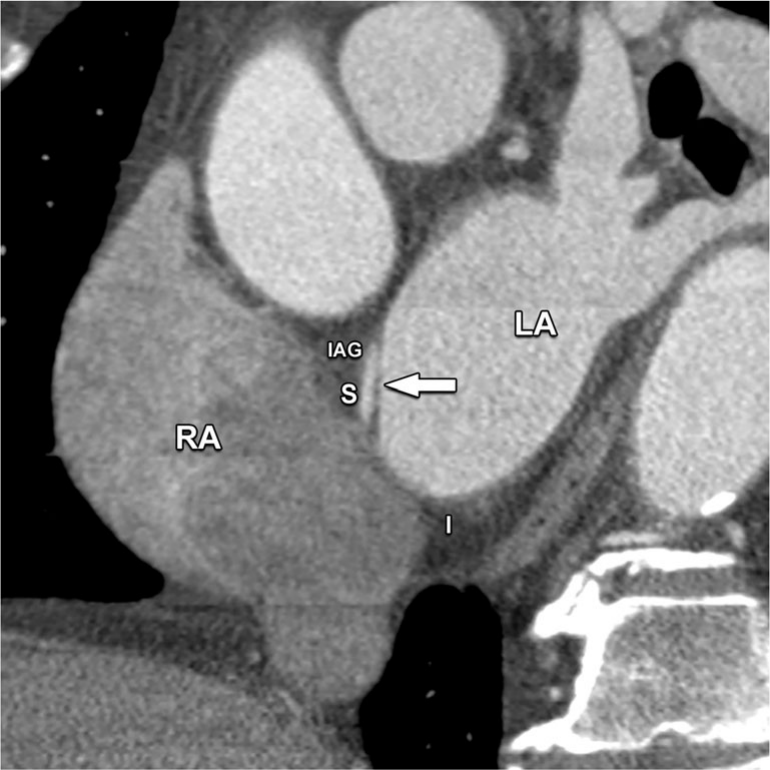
Oblique sagittal post-contrast CT image in a patient with an incidentally found probe PFO at the level of the superior part (S) of the interatrial groove (IAG). Similar to a PFO a septum primum flap (*arrow*) is present. However, in this entity functional closure of the foramen ovale has occurred and hence, no interatrial communication exists, in contrast to a real PFO. *I* inferior part of the interatrial groove, *LA* left atrium, *RA* right atrium
